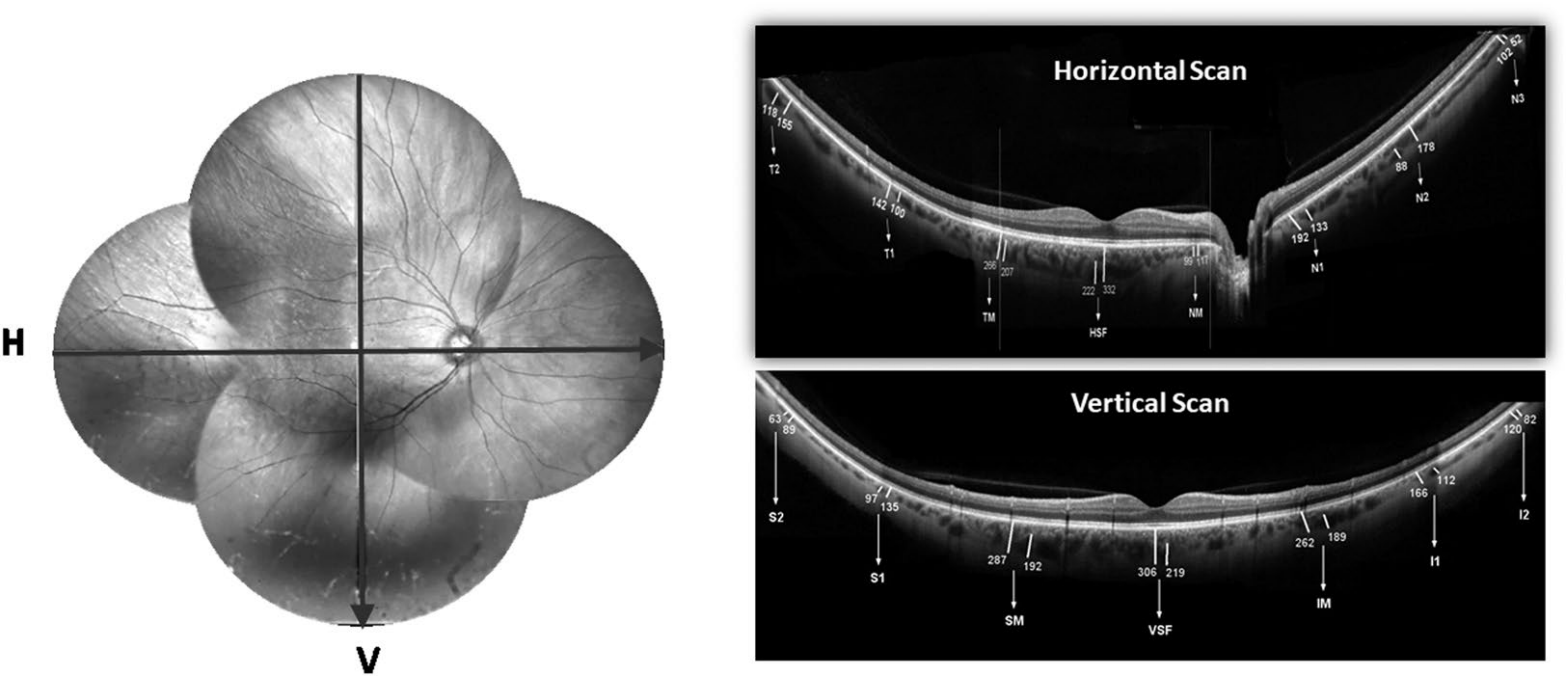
Shows a representative fundus photograph (montage) of right eye with arrows showing the direction of line scans across both horizontal and vertical meridian (left side). Top-right and top-bottom shows representative wide-field optical coherence tomography (WF-OCT) in horizontal and vertical meridian respectively showing the choroidal thickness (CT) and large vessel choroidal thickness (LCVT) in macular segments and all four quadrants. HSF, horizontal subfoveal; VSF, vertical subfoveal; TM, NM, SM, IM represent temporal, nasal, superior and inferior macular points respectively. T1, T2, N1, N2, N3, S1, S2, I1 and I2 are points of measurements in respective quadrants.
